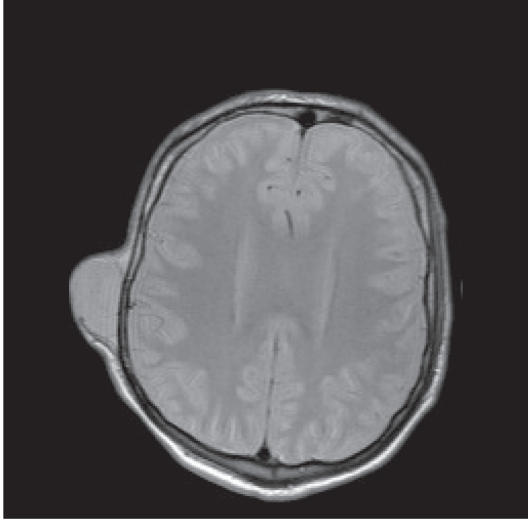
MRI (axial view) showed the subcutaneous temporal
